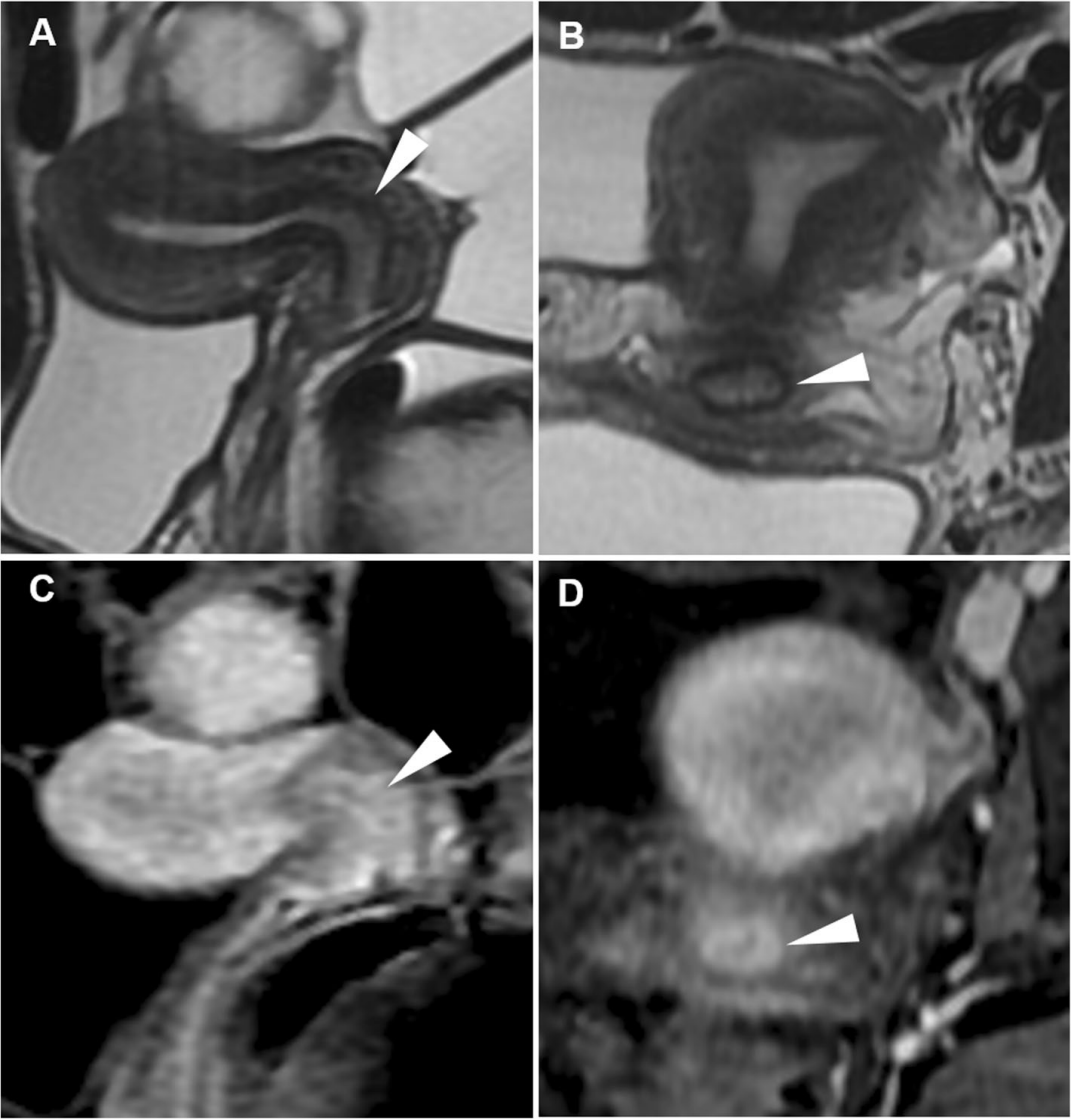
Cervicitis in a 24-year-old woman suffering from pelvic pain. Sagittal (a) and oblique-coronal (b) T2-weighted images show thickening of the mucosal layer at the cervical canal (arrowheads). The corresponding post-gadolinium sagittal (c) and oblique-coronal (d) fat-suppressed T1-weighted images show prominent enhancement along the cervical canal (arrowheads)
